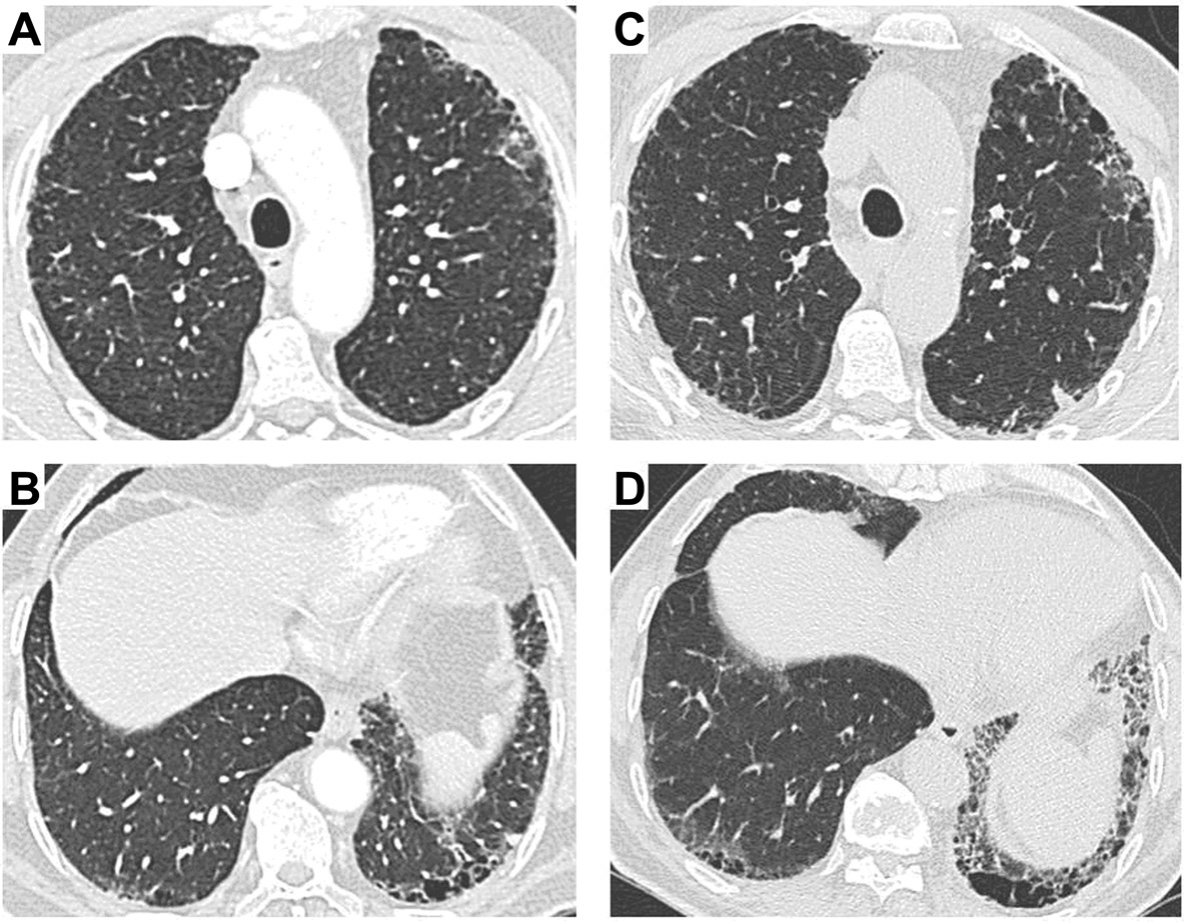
High-resolution computed tomography scan findings in year 2009 (panels a and b) and 2013 (panels c and d). Both examinations revealed sub-pleural reticulation, honeycombing, traction bronchiectasis. Lesions predominated in the lower part of the lungs, and had progressed between 2009 and 2013
